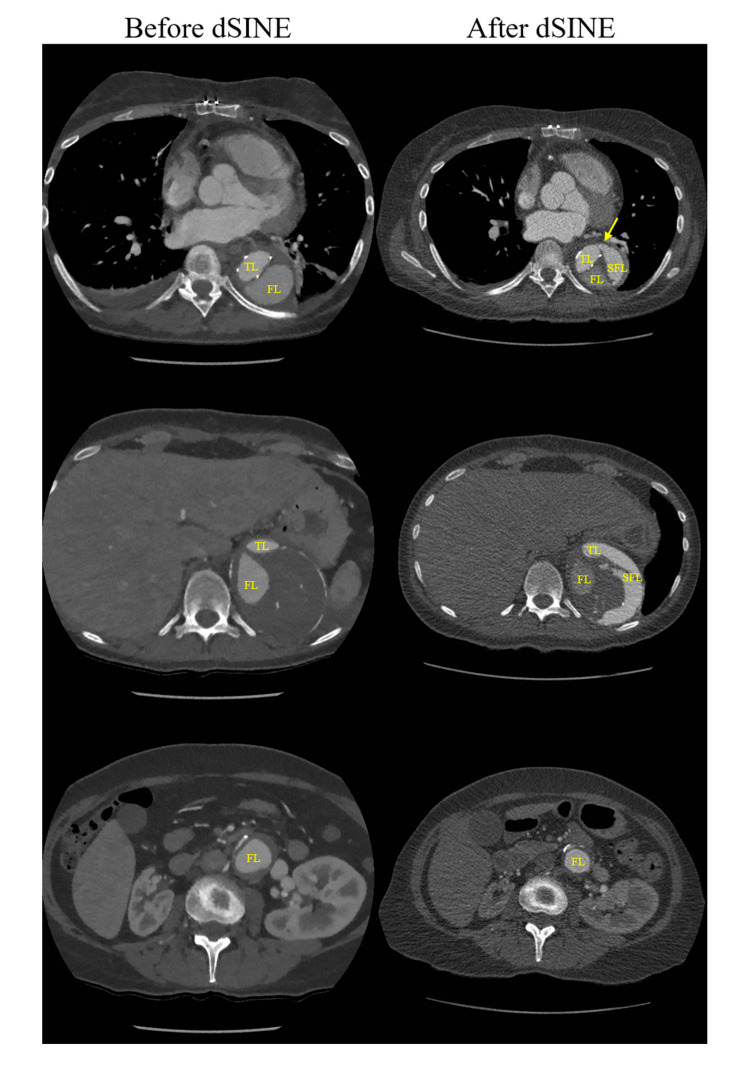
Postsurgical computed tomography angiography images comparing the conformation of the TBAD at different levels before and after the occurrence of the dSINE (marked with a yellow arrow), which determined the formation of a second false lumen (SFL). The true lumen (TL) is completely occluded distal to the emergence of the renal arteries. The initial false lumen (FL) exhibits partial thrombosis.
